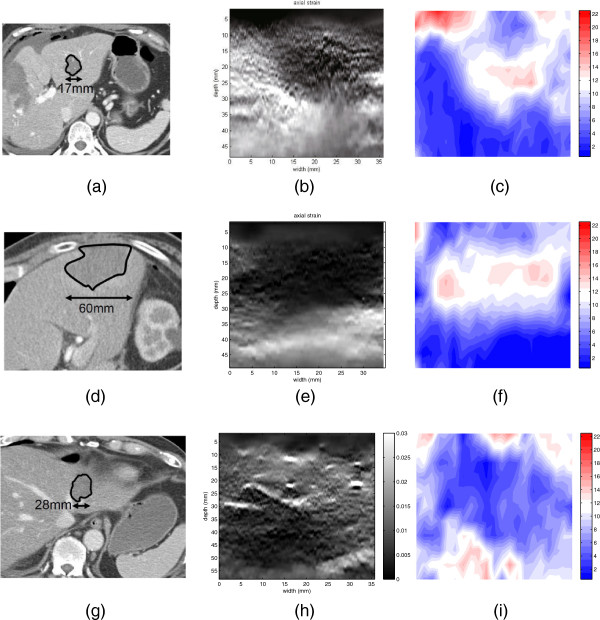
Experiment on clinical data. Clinical data: (a) CT scan of patient 1; (b) strain map of patient 1; (c) our result of patient 1; (d) CT scan of patient 2; (e) strain map of patient 2; (f) our result of patient 2; (g) CT scan of patient 3; (h) strain map of patient 3; (i) our result of patient 3. The non-unity aspect ratio in the axes of the strain images and elasticity images should be considered when comparing them to the CT scans, but the axes of the strain images and elasticity images use the unity aspect ration.
